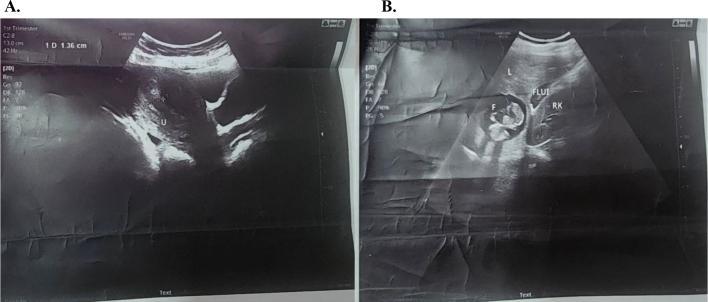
A. Pelvic ultrasound - sagittal plane– showing an empty uterus (labeled U) with a thin endometrium and minimal free posterior cul de sac fluid B. Abdominal ultrasound – longitudinal plane - showing liver (labeled L), fetus (labeled F) of crown-rump length measuring 5.75 cm, free fluid in the Morrison's pouch (labeled Flui) and right kidney (labeled RK).
